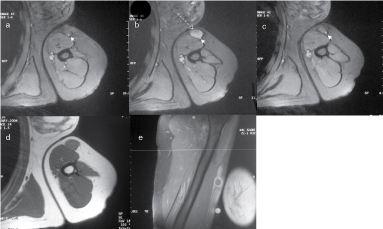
Gradient echo axial MRI from May 2004, above (a), at the level of (b) and below (c) the subcutaneous lesion in the proximal upper arm. The lesion (arrow) is well defined, demonstrates high signal and is closely related to the cephalic vein (arrow heads). *T*
